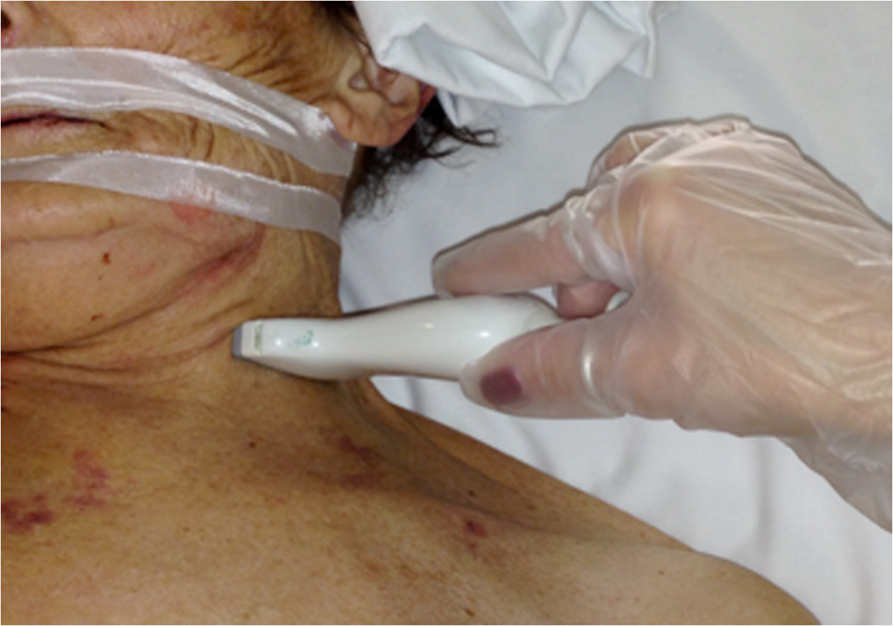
Ultrasound probe position for internal jugular vein detection at the cricoid cartilage level. The patient is in the supine position at 30° with head rotation of 30°.
